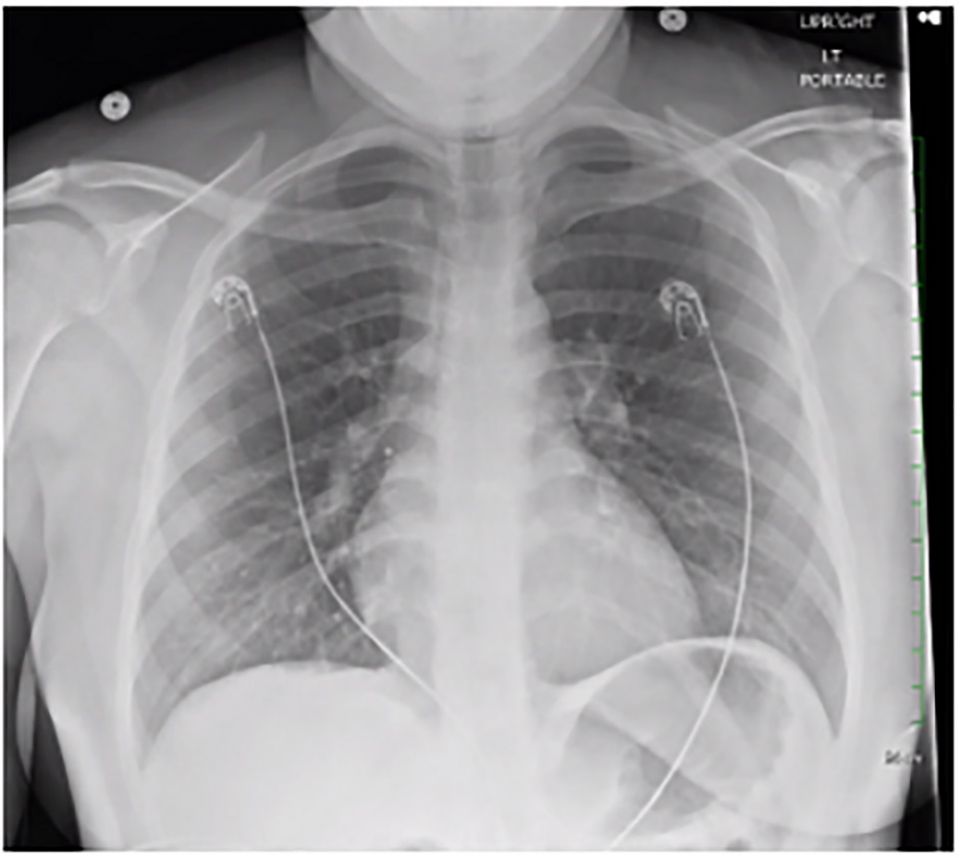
Portable upright anterior-posterior chest radiograph not demonstrating appreciable cardiopulmonary disease.
